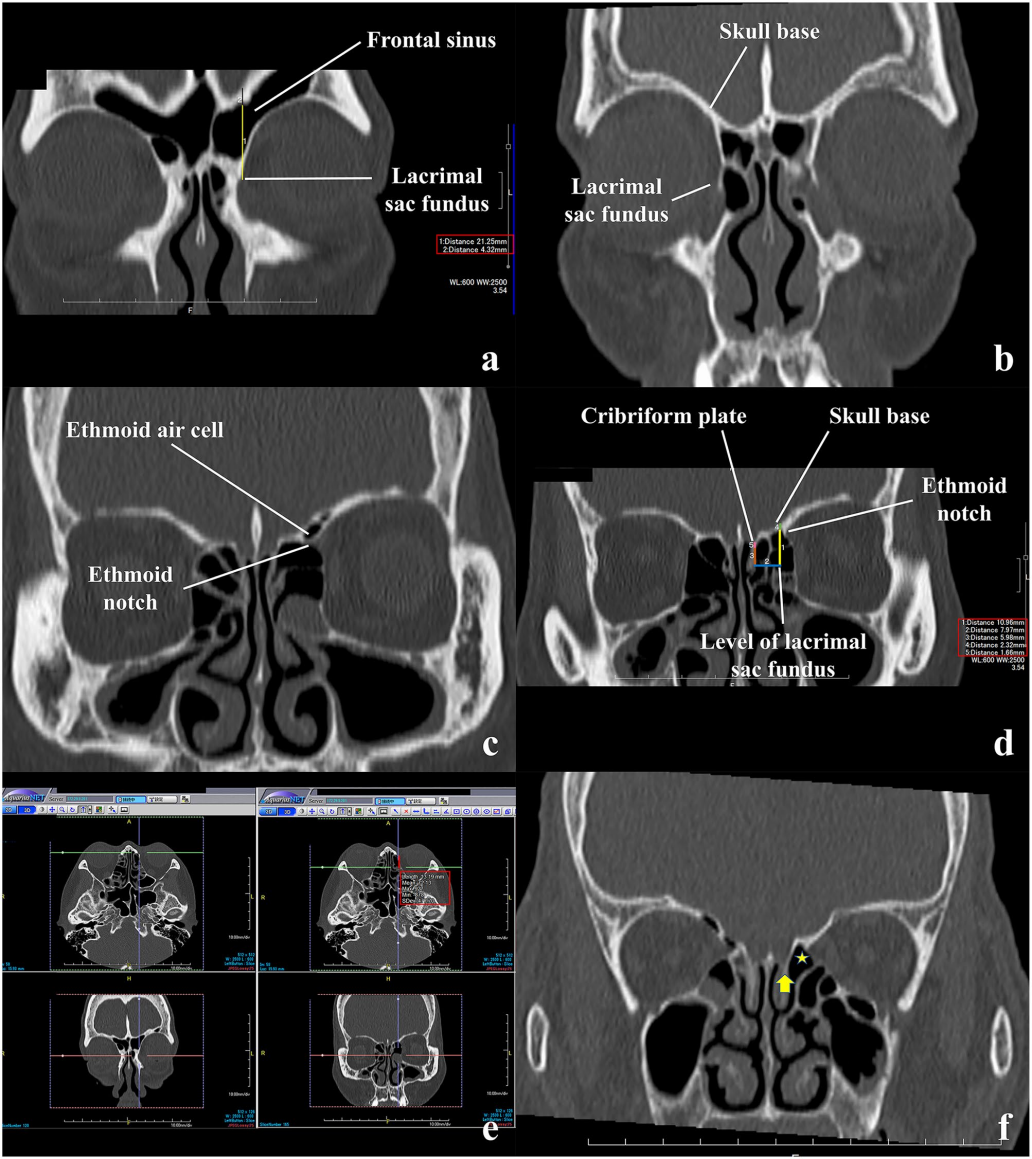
Measurements on computed tomographic (CT) images. The measurement results are surrounded by the red rectangles. (a,b) The coronal CT images showing the lacrimal sac fundus (anterior planes). Measurements of the distance between the lacrimal sac fundus and skull base (#1, yellow line) and the thickness of the skull base (#2, black line) in a patient with interposition of the frontal sinus between the lacrimal sac fundus and skull base (frontal sinus interposition) (a). No frontal sinus interposition (b). (c,d) The coronal CT images with (c) and without interposition of an ethmoid air cell above the ethmoid notch (ethmoid sinus interposition) (d, posterior plane). The vertical distance from the level of the lacrimal sac fundus to the skull base (#1, yellow line), the vertical (#3, brown line) and horizontal (#2, blue line) distances from the level of the lacrimal sac fundus to the lateral margin of the cribriform plate, thickness of the skull base at the vertical level of the lacrimal sac fundus (#4, green line), and that of the cribriform plate were measured (#5, pink line) (d). (e) First, the intersection of the cursor was fixed at the lacrimal sac fundus (left-bottom). The coronal section moved posteriorly until the section showing no ethmoid sinus interposition (right-bottom, posterior plane). The anteroposterior distance of the posterior lacrimal crest from the posterior plane was measured (red line) (right-upper). (f) The cribriform plate (arrow) is positioned below the horizontal level of the lacrimal sac fundus (asterisk).
